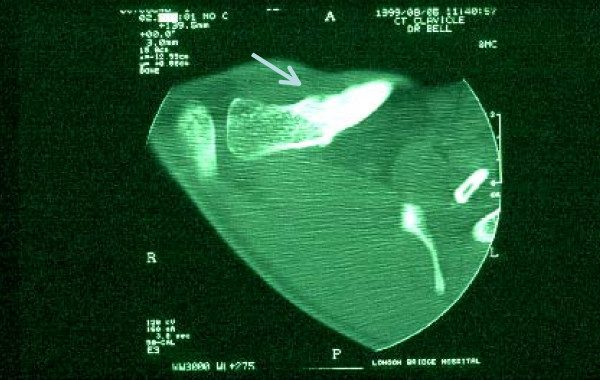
Computed tomography scan of clavicle. Computed tomography slice showing lucent line (arrowed) with adjacent sclerosis consistent with healing stress fracture.
